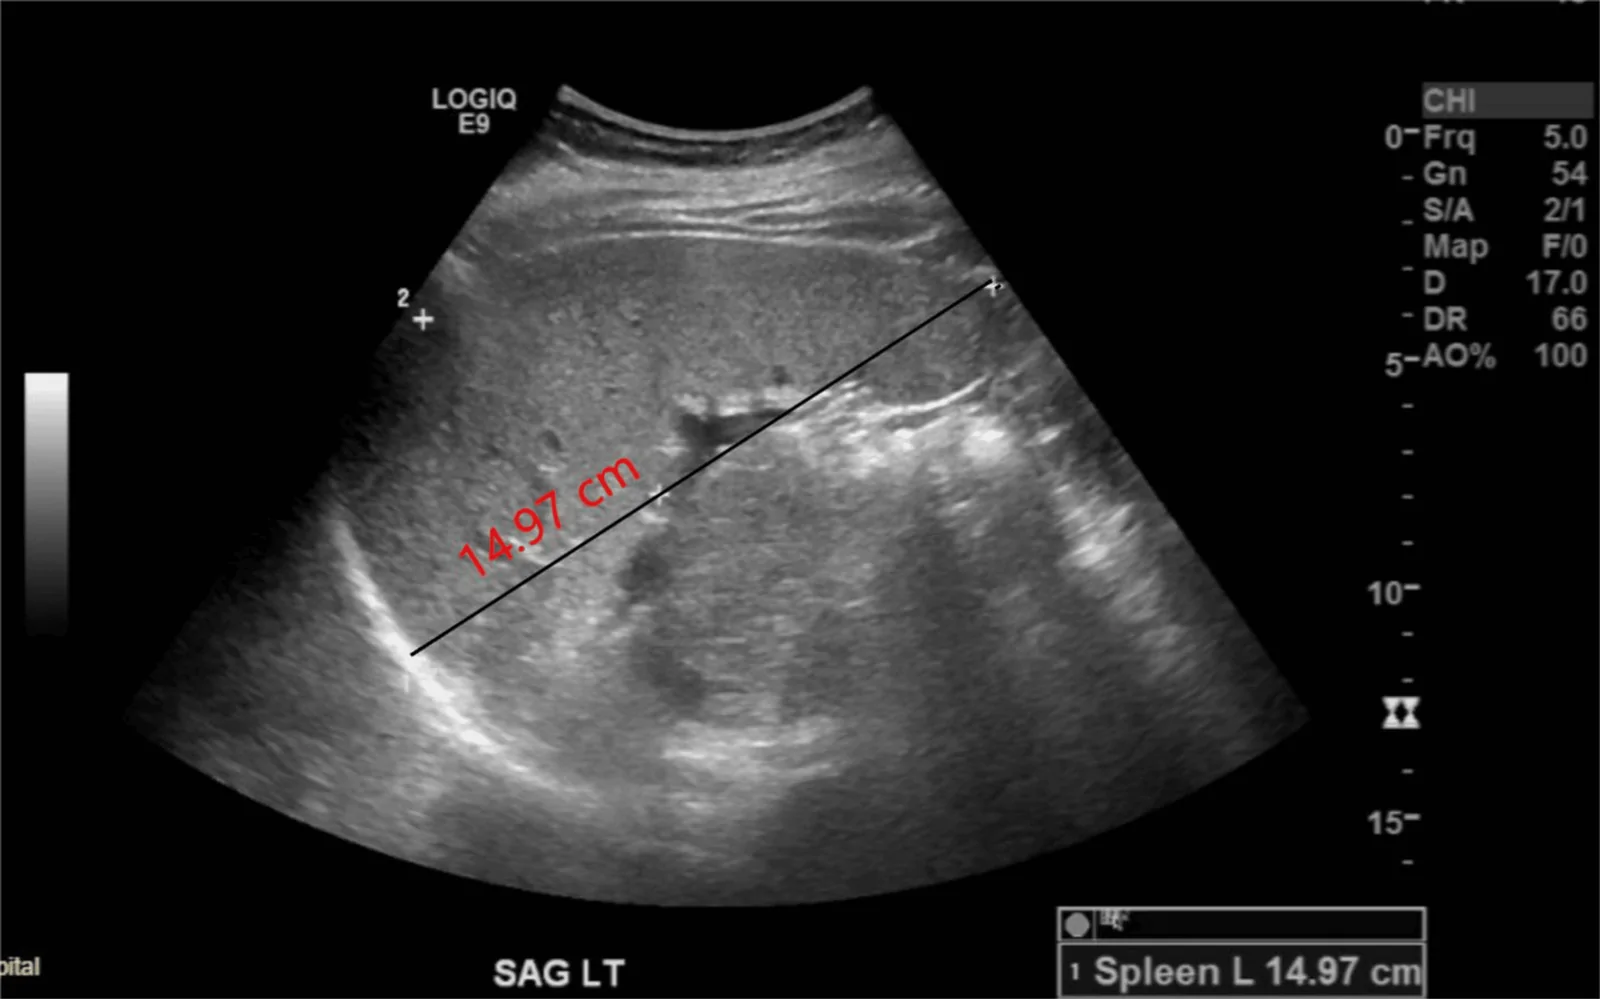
Splenomegaly demonstrated on ultrasound The black line marks the long axis of the spleen, measuring 14.97 cm.
